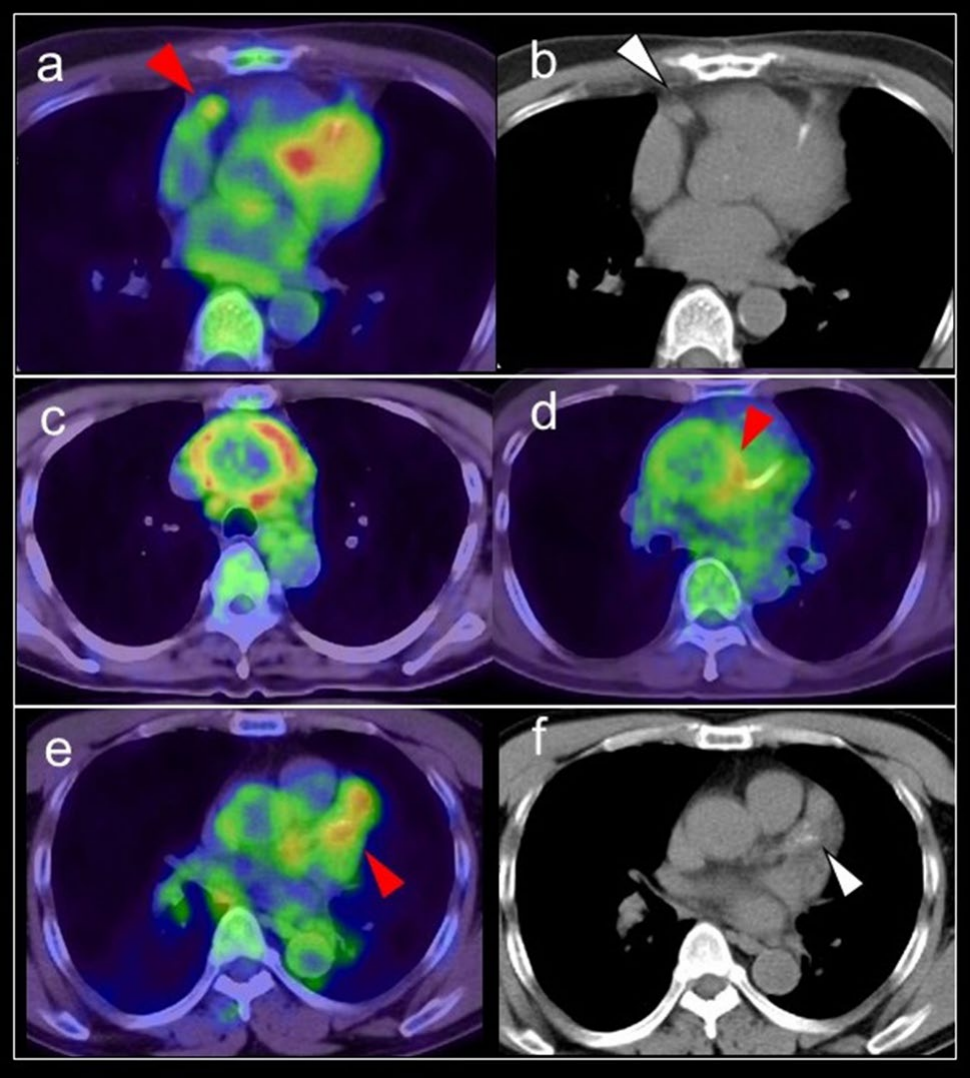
FDG uptake in coronary artery. Incidental findings of FDG uptake in the proximal of right coronary artery (a red arrowhead), suggesting the active plaque in the artery (b white arrowhead). The patient with Takayasu arthritis showed intense FDG uptake at ascending aorta (c), and the uptake reached the origin of right coronary artery (d). FDG uptake (e red arrowhead) in swollen left coronary artery (f white arrowhead) was diagnosed involvement of IgG4 related disease in coronary artery representing perivascular immuno-inflammation
